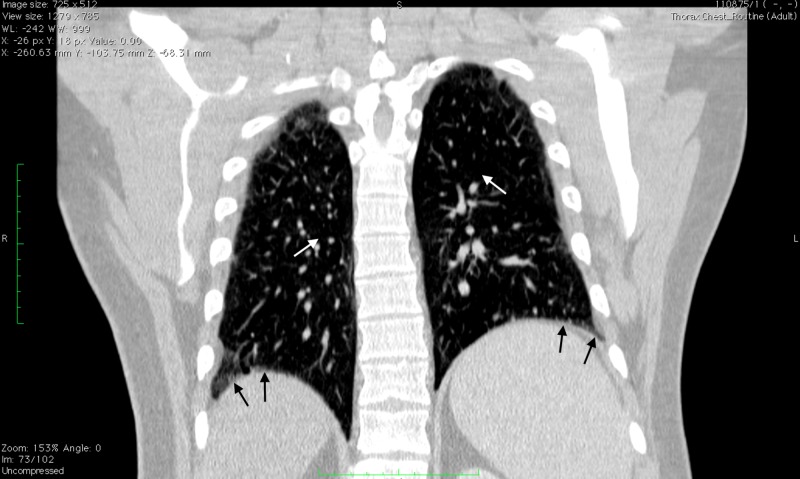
Multidetector Helical Computed Tomography Scan of the Chest, Coronal View This image displays a coronal view of our patient with stable emphysematous changes bilaterally shown by the white arrows and bilateral lower lobe dependent atelectasis shown by the black arrows.
